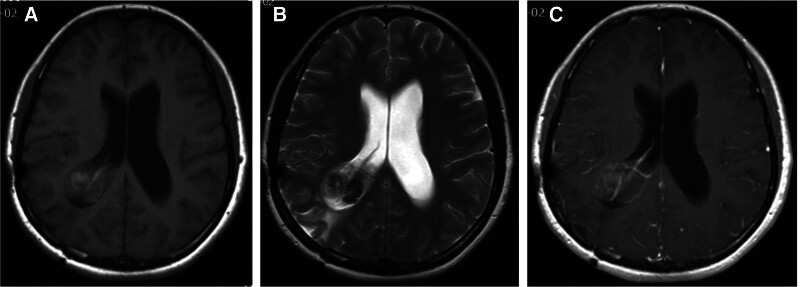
MRI images showing the PXA in the lateral ventricle. Axial T1W1 sequence shows a hypointense right lateral ventricle mass with patchy hyperintense signals (A). The mass shows mixed signals on T2WI sequence (B). Heterogeneous enhancement is seen on T1WI contrast-enhanced sequence (C). PXA = pleomorphic xanthoastrocytoma.
